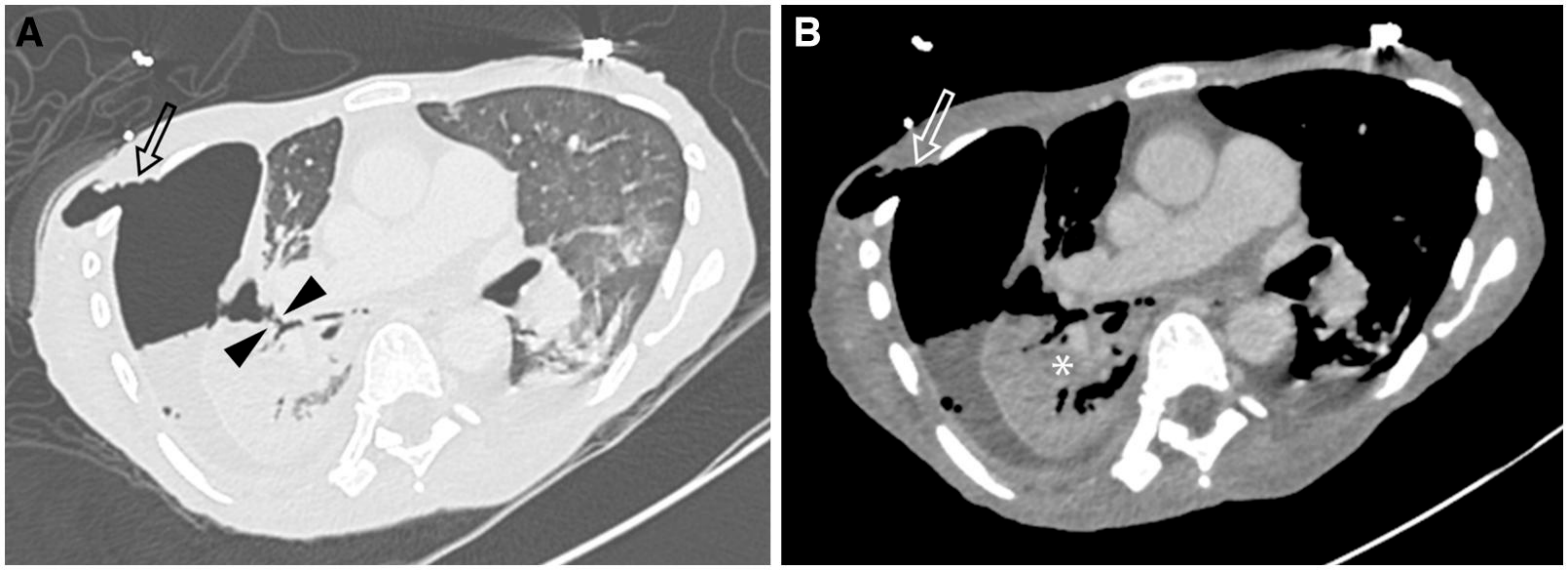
Right empyema necessitans with bronchopleural fistula in a 47-year-old woman with disseminated TB. Axial contrast-enhanced CT images obtained in (A) lung and (B) soft tissue windows show the fistulous tract (arrowheads in A) between right lower lobe bronchus and empyema in right pleural space with extension into the chest wall (arrows in A and B). Soft tissue setting image better shows the right hydropneumothorax with associated smooth pleural thickening and heterogeneous enhancement of the atelectatic right lower lobe (asterisk in B), indicating pneumonia in the atelectatic lung. Small left pleural effusion is also seen.
